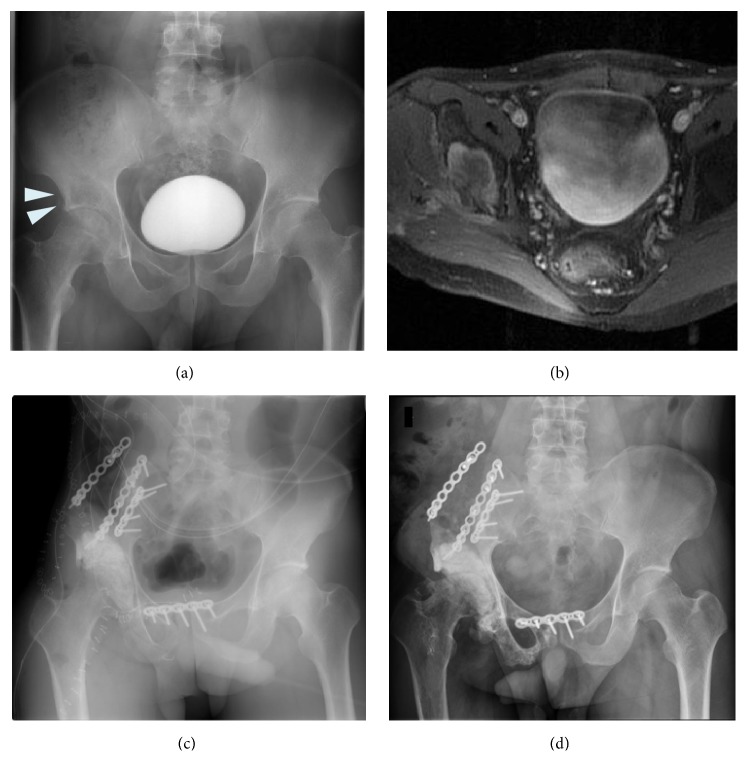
(a) Preoperative anteroposterior radiograph of the pelvis of a 38-year-old man who had MFH of bone in the acetabulum. (b) Gadolinium-enhanced axial T1-weighted MRI showing the tumor arising in the acetabulum. (c) Postoperative radiograph after reconstruction using a frozen bone autograft. (d) Follow-up radiograph 1.5 years after surgery showing osteoarthritis of the hip joint.
